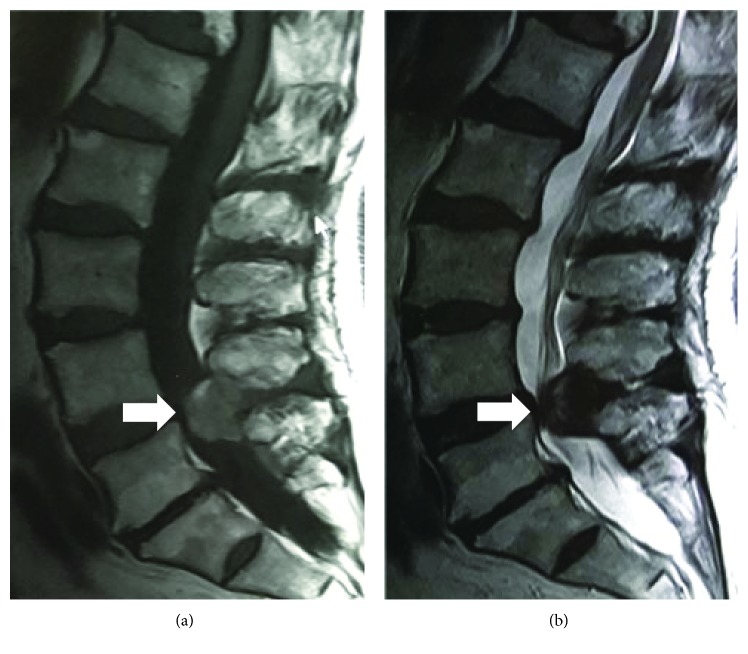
Magnetic resonance images showing a posterior epidural mass at L4/5 compressing the thecal sac and spinal cord and linking with the ligamentum flavum.
